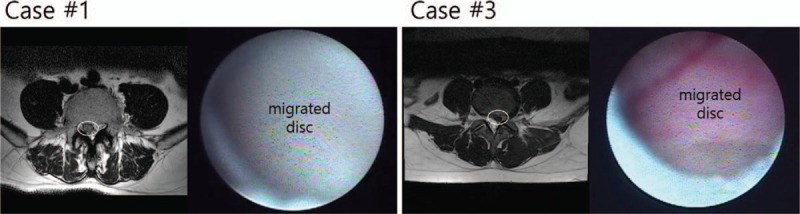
Migrated disc of Case 1 and 3 shown in MRI and epiduroscope. White circles in MRI images indicates the migrated disc. MRI = Magnetic resonance imaging.
